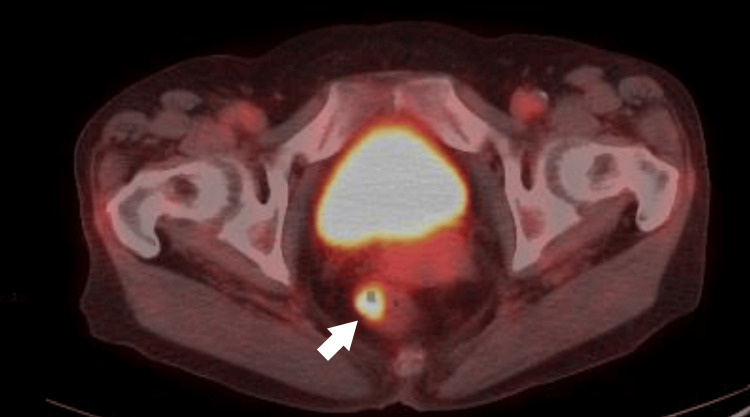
Fluorodeoxyglucose (FDG)-PET/CT image showing intense uptake in the rectal lesion (SUVmax: 10.59, arrow).
